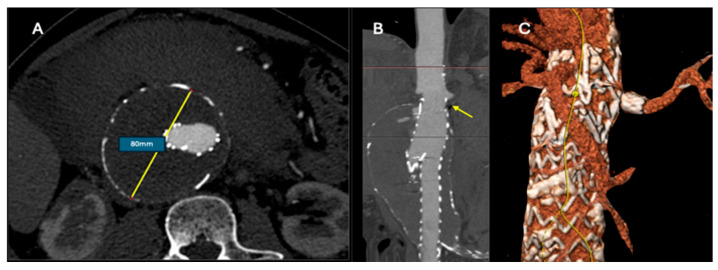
PG-EVAR for ruptured AAA after previous EVAR. Eleven patients were managed with PG-EVAR. Among them, a patient with an 80 mm juxta-renal aneurysm after previous EVAR was treated with a single parallel graft targeting the preservation of the left renal artery (A,B). The short infrarenal neck did not permit the application of standard EVAR ((B); yellow arrow). The 36-month CTA confirmed the favorable technical outcome with no endoleak and a patent target vessel (C).
